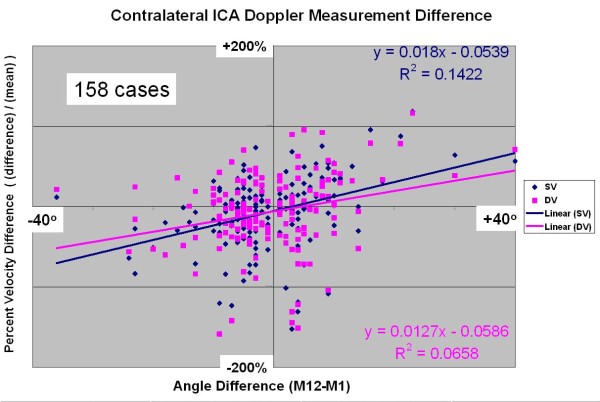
Percent Velocity Value Change with Doppler Angle Change.
